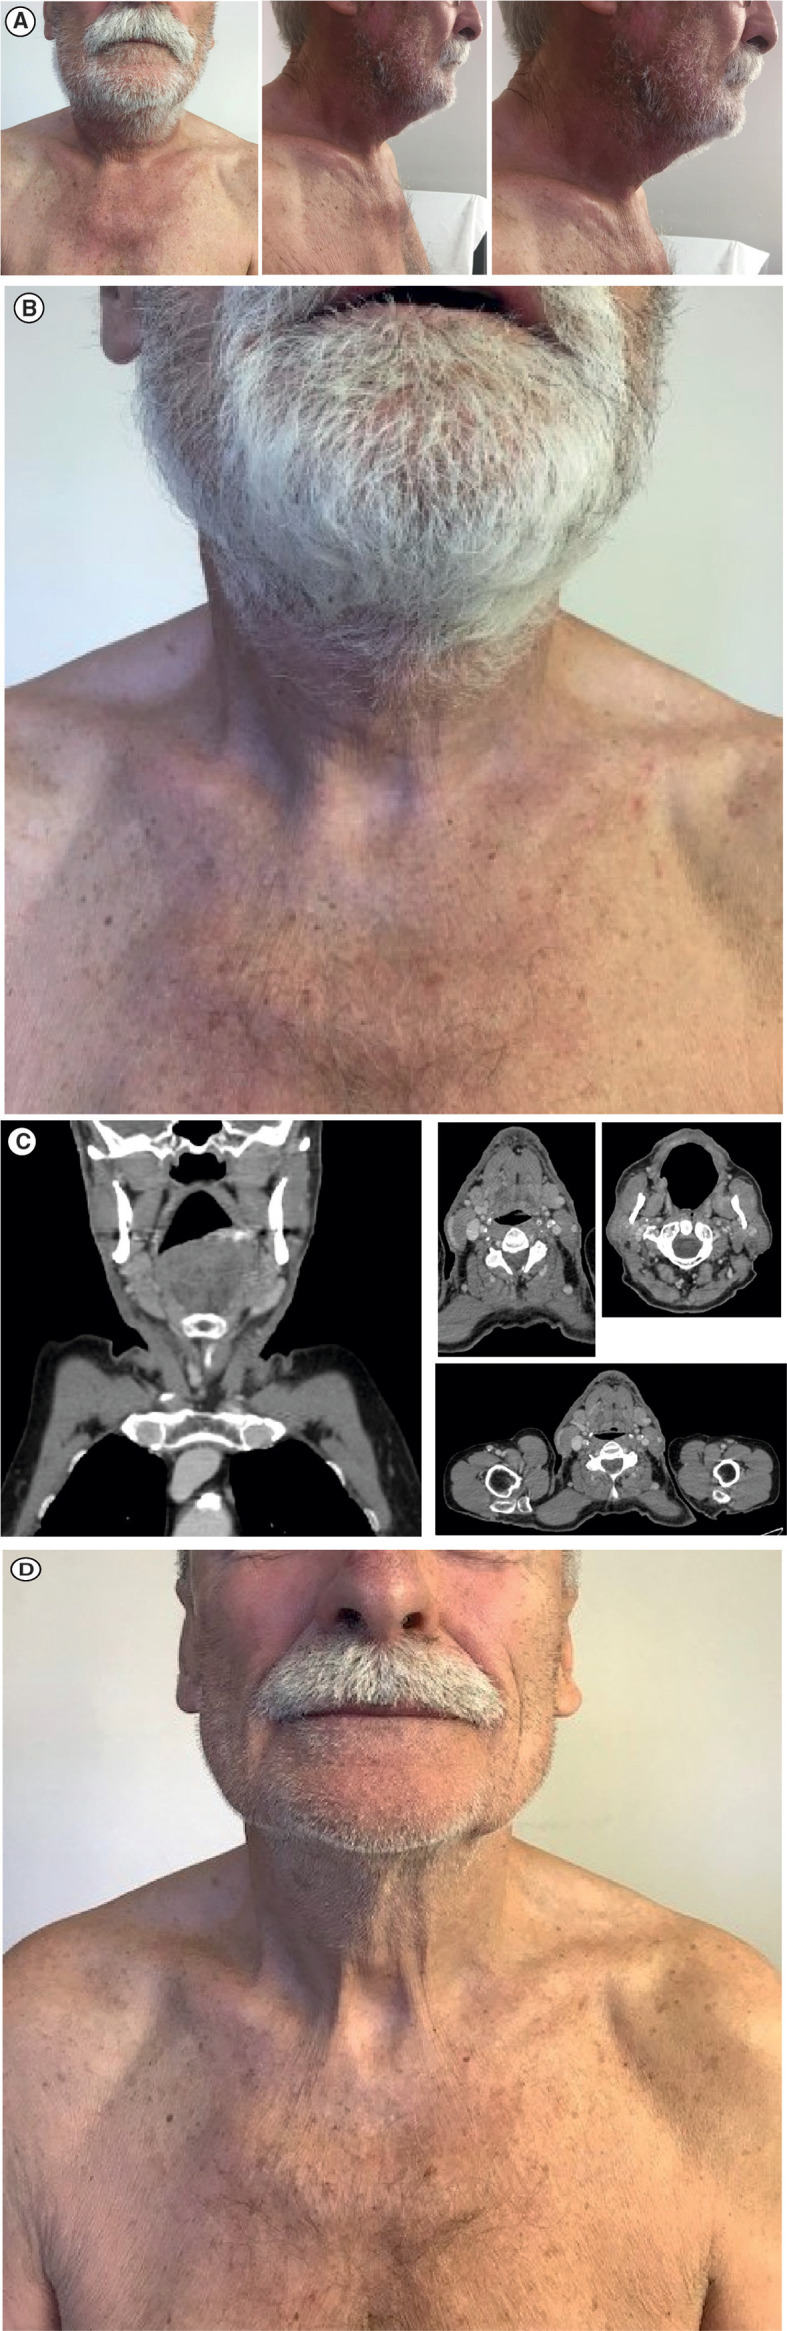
Response to avelumab treatment: (A) Clinical presentation of substantial measurable reduction in lesion size after one dose of avelumab. (B) Clinical presentation of confirmed complete regression of the tumor mass after three doses of avelumab. (C) Face and neck coronal (leftmost panel) and axial (right panels) CT scans showing complete radiological response. (D) Clinical presentation of ongoing complete response on 30 October 2019. CT, computed tomography.
